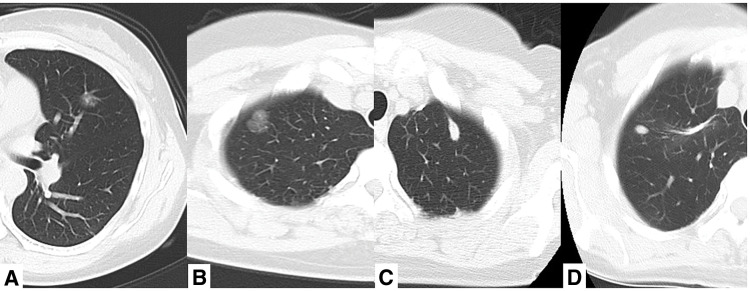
Pulmonary metastasis of adenoid cystic carcinoma. Chest CT showed GGNs or solid nodules. At present: 14-mm GGN in the S4 of the left lung (A); 7 years ago: 16-mm GGN in the S1 of the right lung (B); 3 years ago: 13-mm solid nodule in the S1+2 of the left lung (C); 1 year ago: 8-mm solid nodule in the S1 of the right lung (D). GGN, ground-glass nodule
